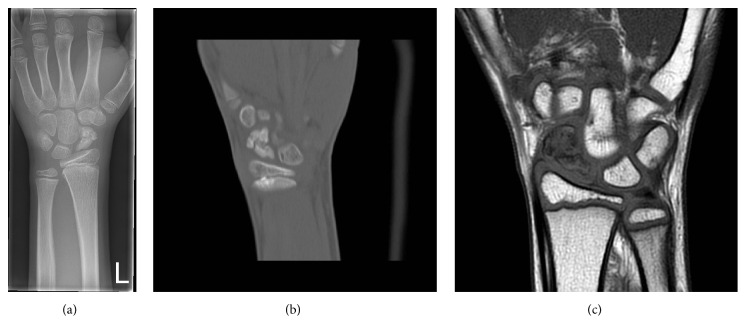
(a) Plain radiograph at acute presentation showing a fracture of the scaphoid waist with sclerosis, central cystic bone alteration, and deformity of the proximal pole. (b) CT scan 1 week after the initial injury showing a more pronounced central cystic bone alteration and irregularity of the fracture border. (c) MRI T1 showing signal loss of the whole scaphoid.
